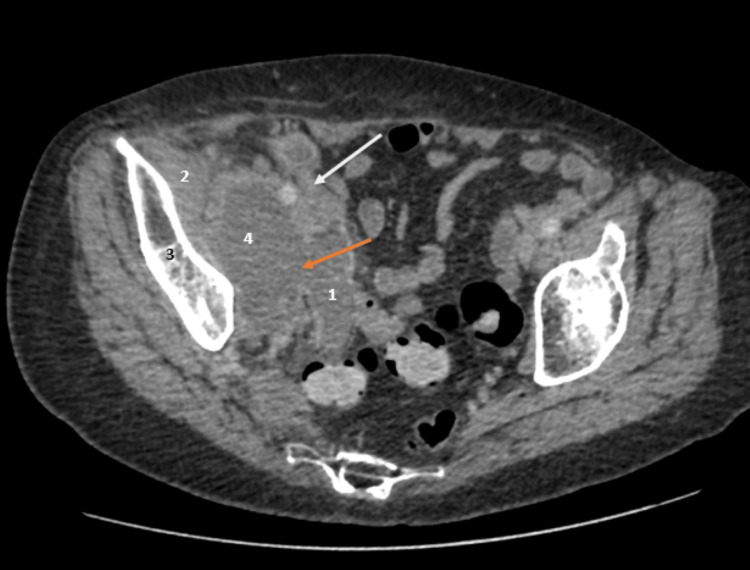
Contrast-enhanced computed tomography abdomen and pelvis axial view 1. Dilated appendix, 2. Iliacus muscle, 3. Pelvis, 4. Psoas abscess White arrow: Base of appendix, Orange arrow: Ruptured appendix communicating with abscess
